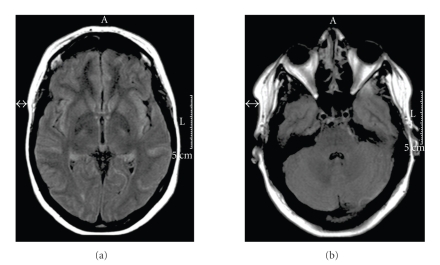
A followup MRI examination three months after admission shows a complete resolution of the vasogenic edema.
